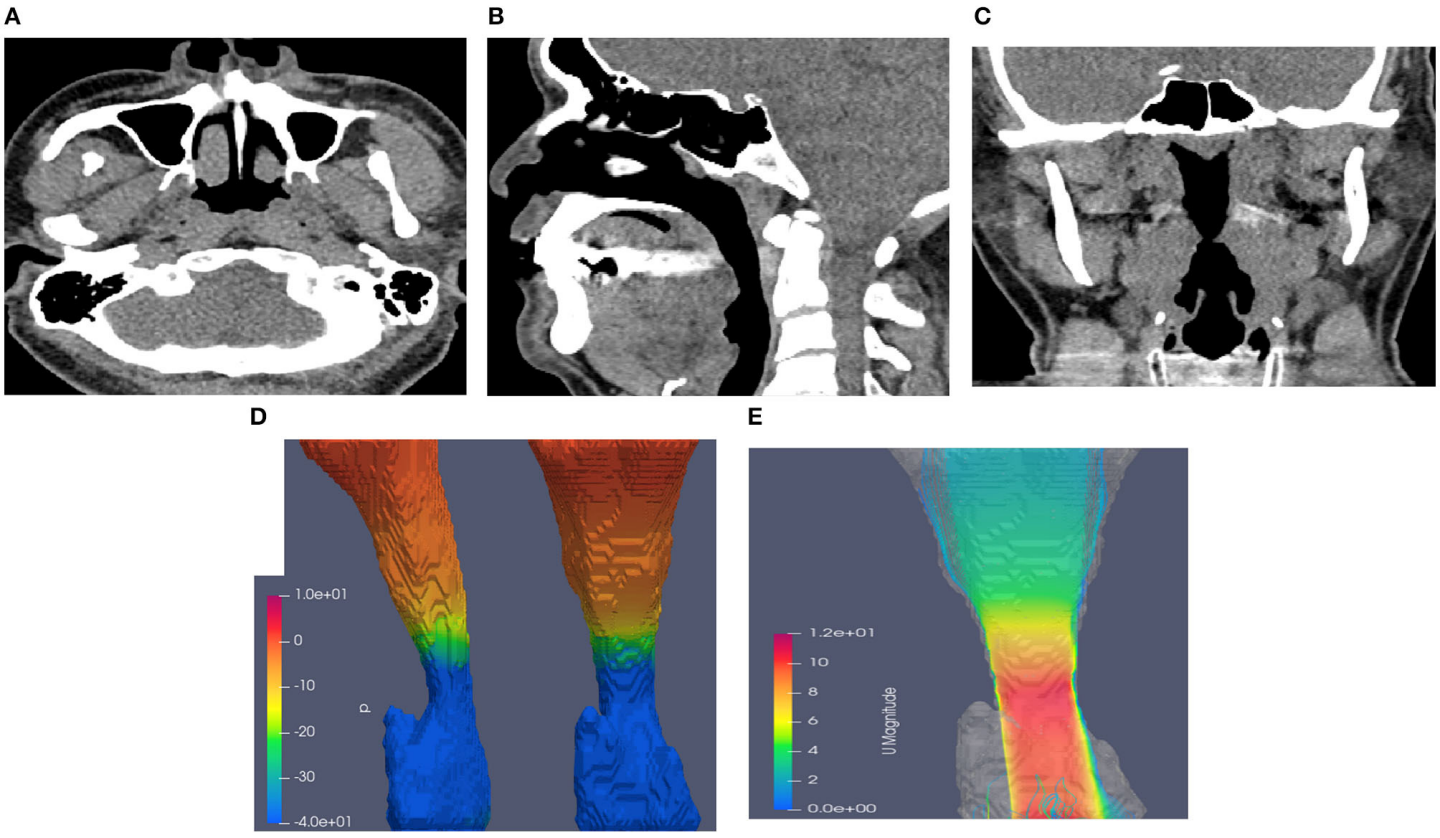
Neck CT with computational fluid dynamics study of the 44-year-old male showed CTL34. (A) Axial view. (B) Sagittal view. (C) Coronal view. (D) Airway pressure of 51.88 Pa. (E) Airflow velocity of 10.7 m/s.
